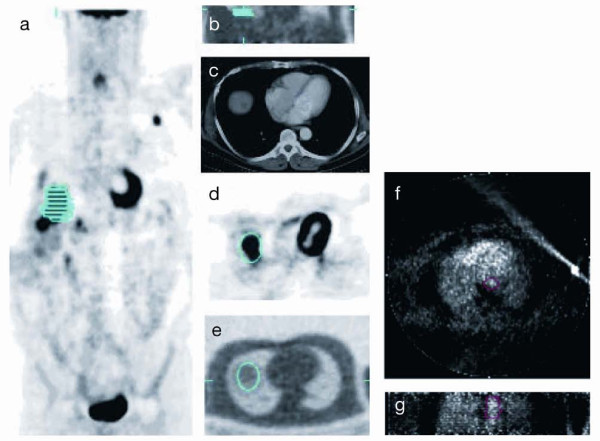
Reference tumour volume of interest using the attenuation image for the FDG and ^15^O-water studies. Liver metastasis defined on the FDG-PET emission image ((a) coronal and (d) transaxial), also seen on the CT scan (c), was identified and transposed onto the ^15^O-water acquired attenuation image ((b) coronal and (e) transaxial) to be subsequently applied to the dynamic ^15^O-water emission image. The descending aorta volume of interest markup from ^15^O-water early dynamic frames ((f) transaxial and (g) coronal).
